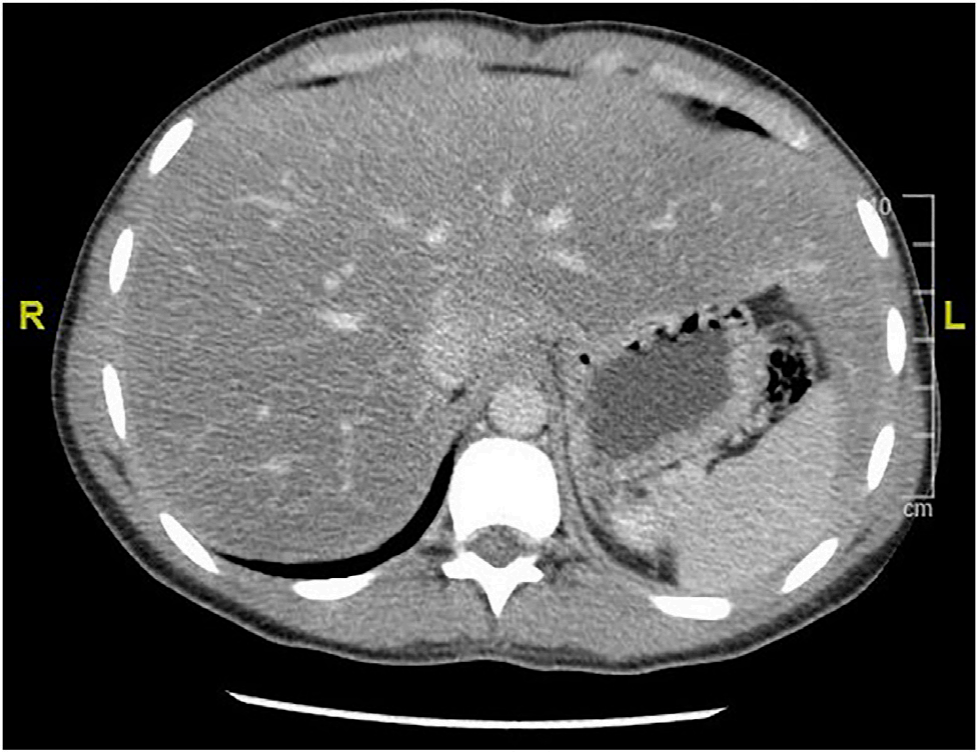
CT imaging features of liver steatosis. Caption: Transverse CT image of the liver showing decreased density of the liver compared to the spleen in this 38-year old patient with NAFLD. In GlyH, the inverse image can be witnessed with increased density compared to the spleen, but due to concomitant steatosis, this contrast is potentially attenuated in metabolic patients.
